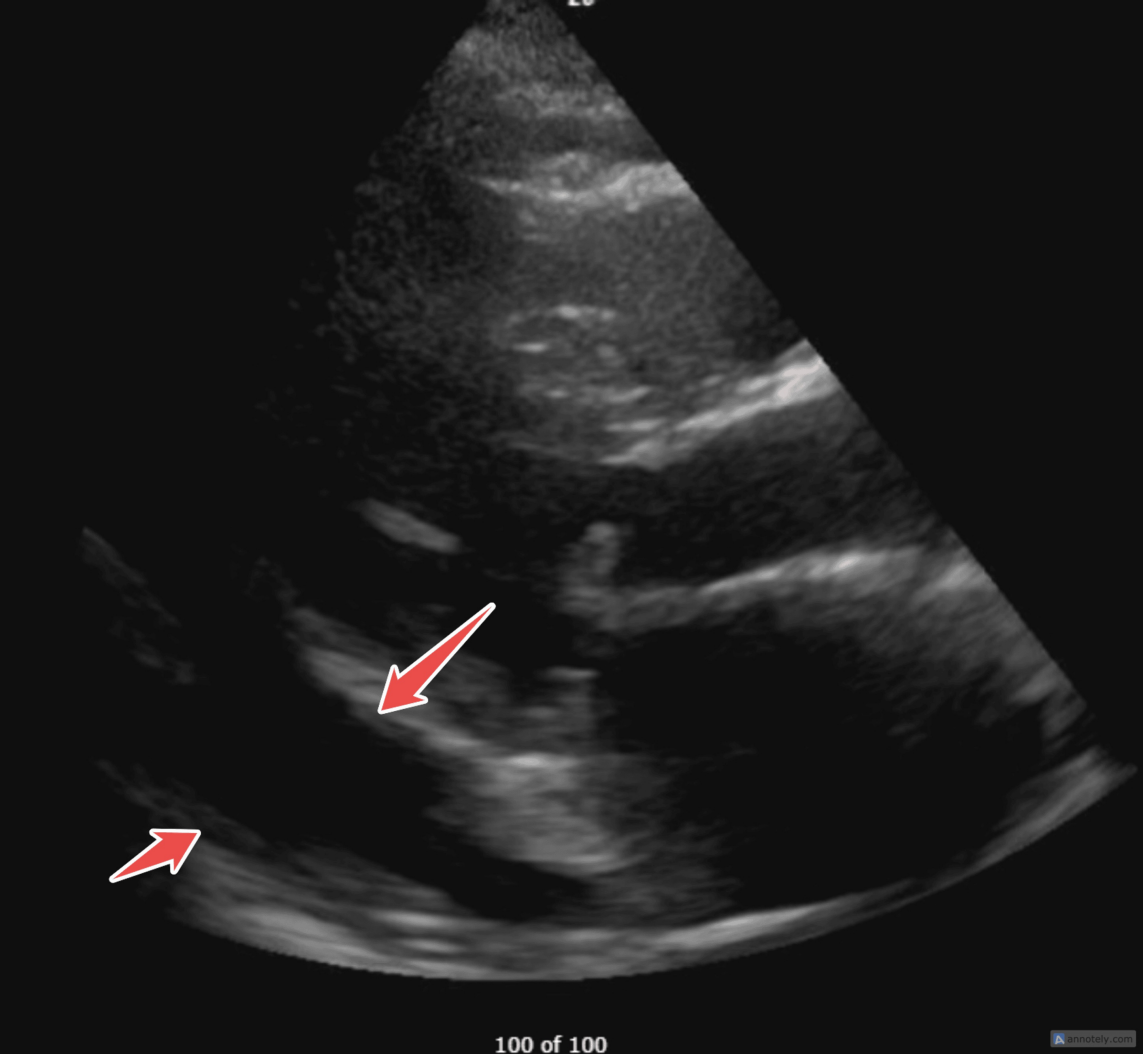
TTE showing severe pericardial effusion TTE: transthoracic echocardiogram
